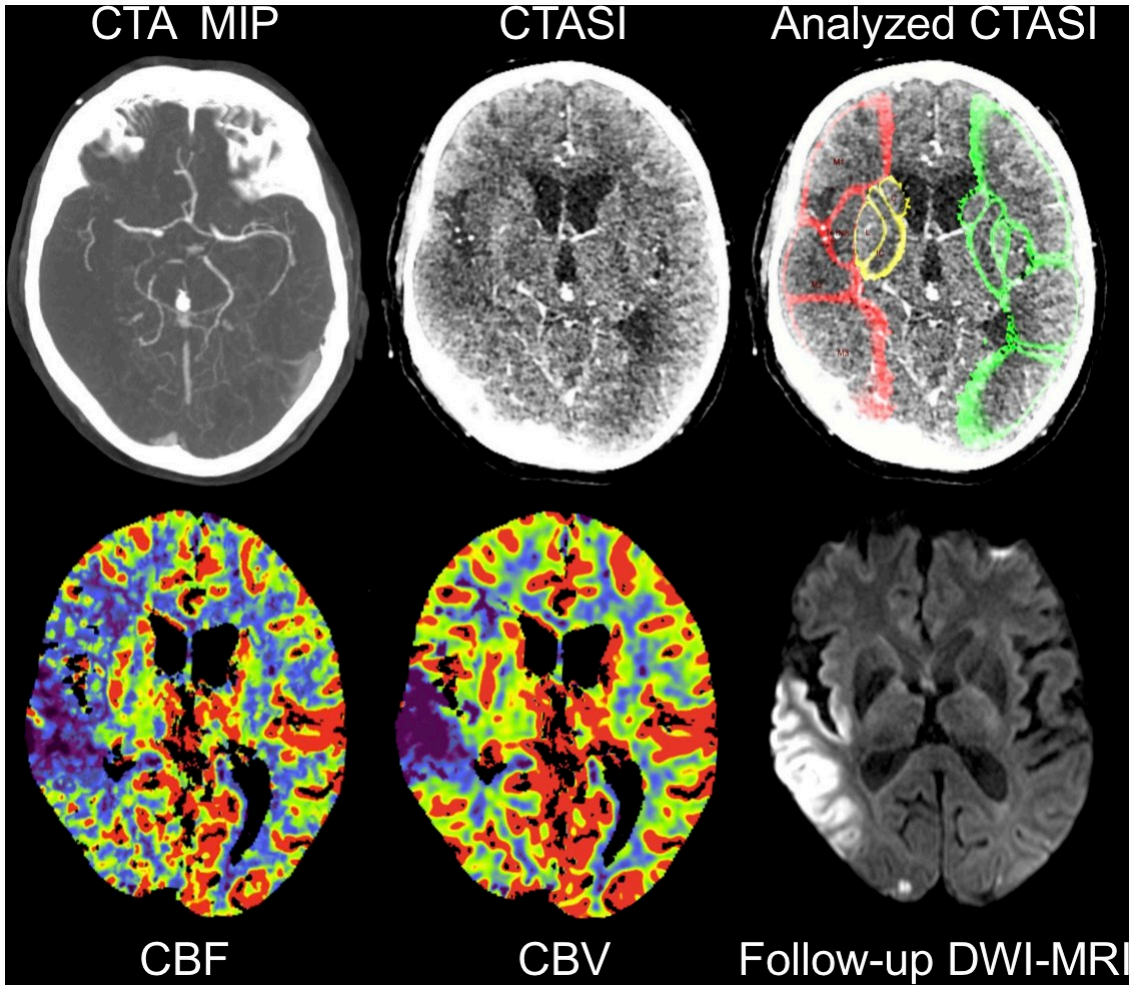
Case example of a 76-year-old male patient. CTA MIP shows a proximal M1 occlusion on the right side with consecutive cerebral blood flow and blood volume deficit on CTP. Noncontrast CT ASPECTS on admission was interpreted as 8 by both readers (not displayed). CTASI ASPECTS was 7 for reader 1 and 8 for reader 2. CTA source images without and after automated segmentation and attenuation measurements in ASPECTS regions presenting deficit of contrast uptake in the affected regions. Colors indicate reduction in regional x-ray attenuation compared with the contralateral hemisphere (green = difference < 1 HU; yellow = difference ≥ 1 and < 3 HU; red = difference > 3 HU). Notably, the analysis provides good match with ischemic hypoperfusion on CBF maps but overestimates infarction core on CBV and final infarction displayed on follow-up diffusion-weighted MRI after complete recanalization (mTICI 3). Abbreviations: CTA, CT angiography; MIP, maximum intensity projection; CTP, CT perfusion; CBF, cerebral blood flow; CBV, cerebral blood volume; DWI, diffusion-weighted imaging; ASPECTS, Alberta Stroke Program Early CT Score; mTICI, modified Treatment in Cerebral Ischemia Score.
